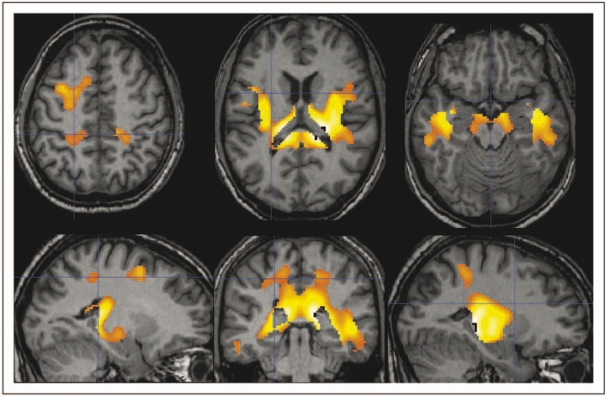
Axial sections (first row, patient’s left side is on the observer’s left side), sagittal sections of the left (bottom left) and right (bottom right) hemispheres, and coronal section (bottom row, centre) from selected levels of a normal subject T1-weighted volume (in greyscale). The regions of significant white matter loss in patients with multiple sclerosis as compared with controls are overlaid in colourscale. Bilaterally, in the periventricular regions in the temporal lobes, juxtacortical insular regions, extending posteriorly through the internal capsule to the thalami, and the splenium of the corpus callosum are involved.
